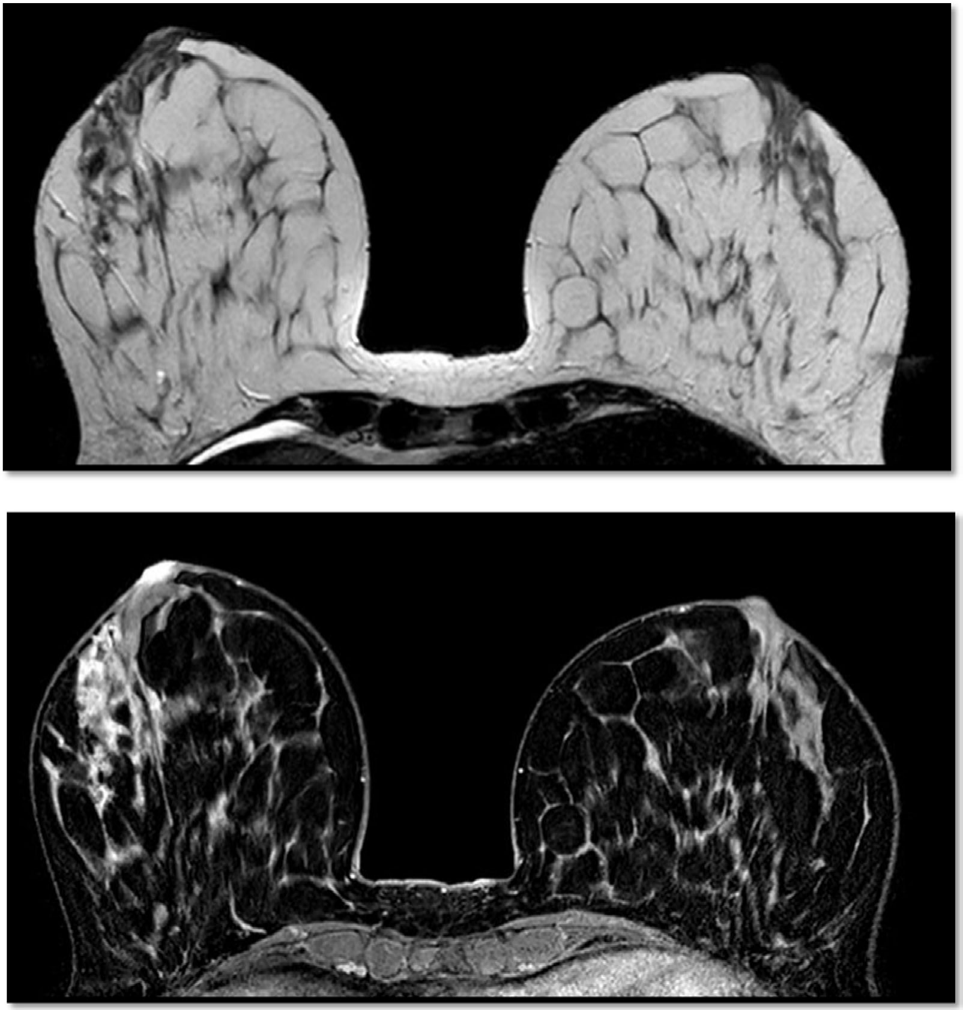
Breast magnetic resonance imaging revealed several multicentric tumoral lesions of the right breast with extension to the nipple–areola complex.
